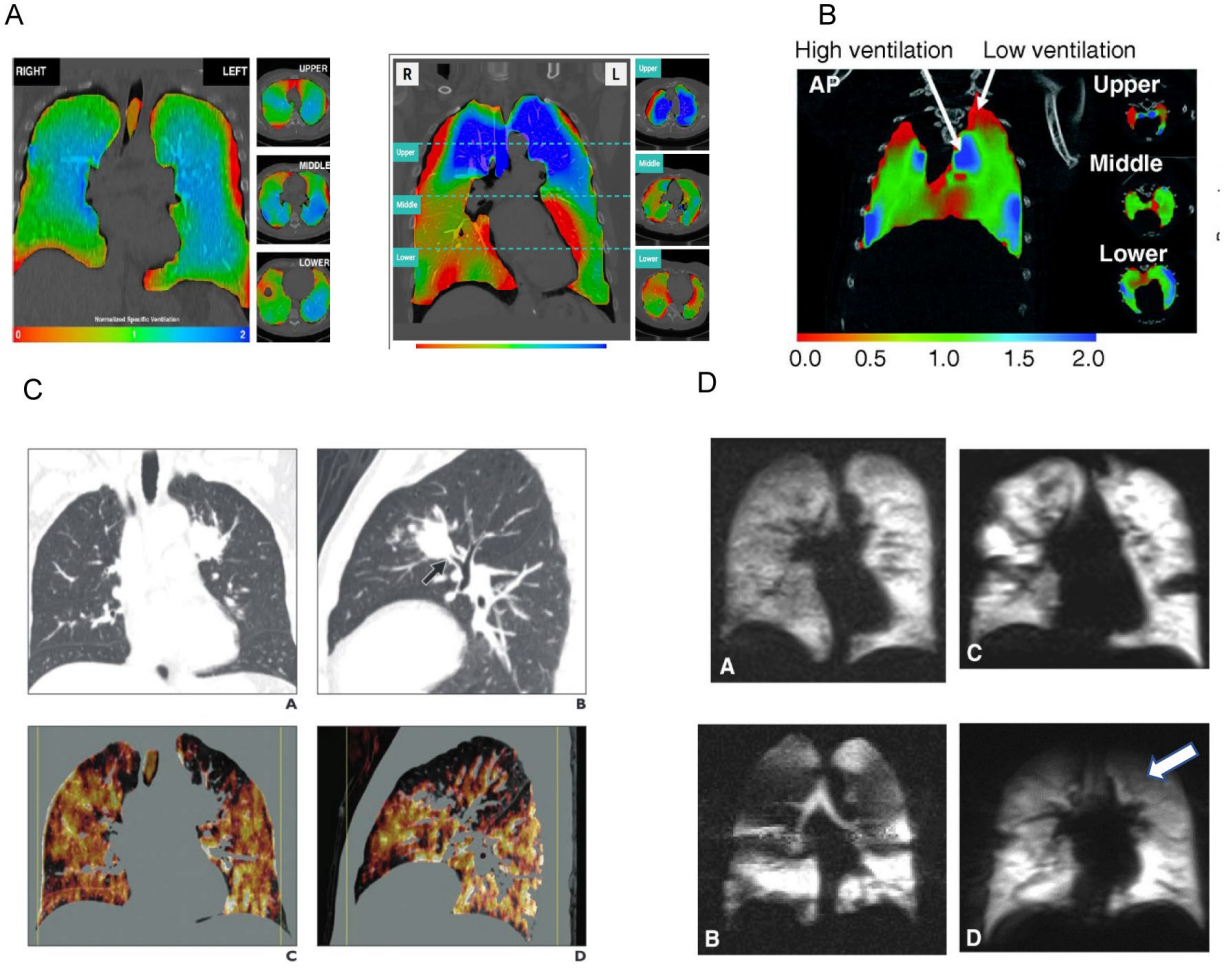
Examples of radiographically derived ventilation. Panel (A) shows XV analysis of regional lung ventilation using a color scale (red represents areas with decreased ventilation) (31). Panel (B) represents 4D CT imaging which shows regional areas of high ventilation and areas of low ventilation (red represents areas with decreased ventilation). Reprinted with permission of the American Thoracic Society. Copyright © 2023 American Thoracic Society. All rights reserved. The American Journal of Respiratory Cell and Molecular Biology is an official journal of the American Thoracic Society. (32). Panel (C) shows Xenon CT imaging with heterogenous distribution of Xenon due to this patient’s emphysematous disease. Ventilation defects are noted in the left upper lobe (white arrow). Adapted from the American Journal of Roentgenology, Copyright © 2014, copyright owner as specified in the American Journal of Roentgenology. (19). Panel (D) shows function MRI images with hyperpolarization of ^3^He/^129^Xe. Regional hypoventilation is noted by white arrows (33).
